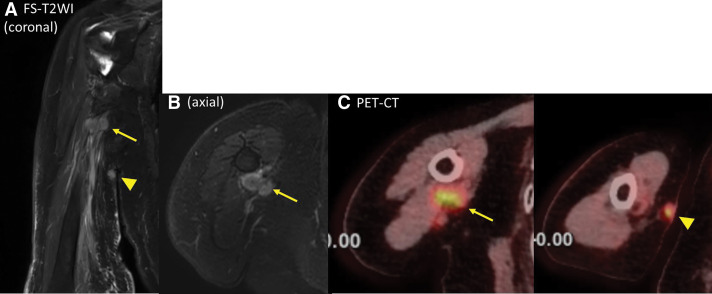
(A, B) MRI (fat-suppressed T2-weighted imaging) scans taken 1 year and 3 months after the initial surgery. (C) PET-CT scan taken 1 year and 3 months after the initial surgery. A mass was observed at the tumor resection site (arrow), and skin metastasis was also identified (triangle). T2WI, T2-weighted imaging; PET-CT, positron emission tomography-computed tomography
